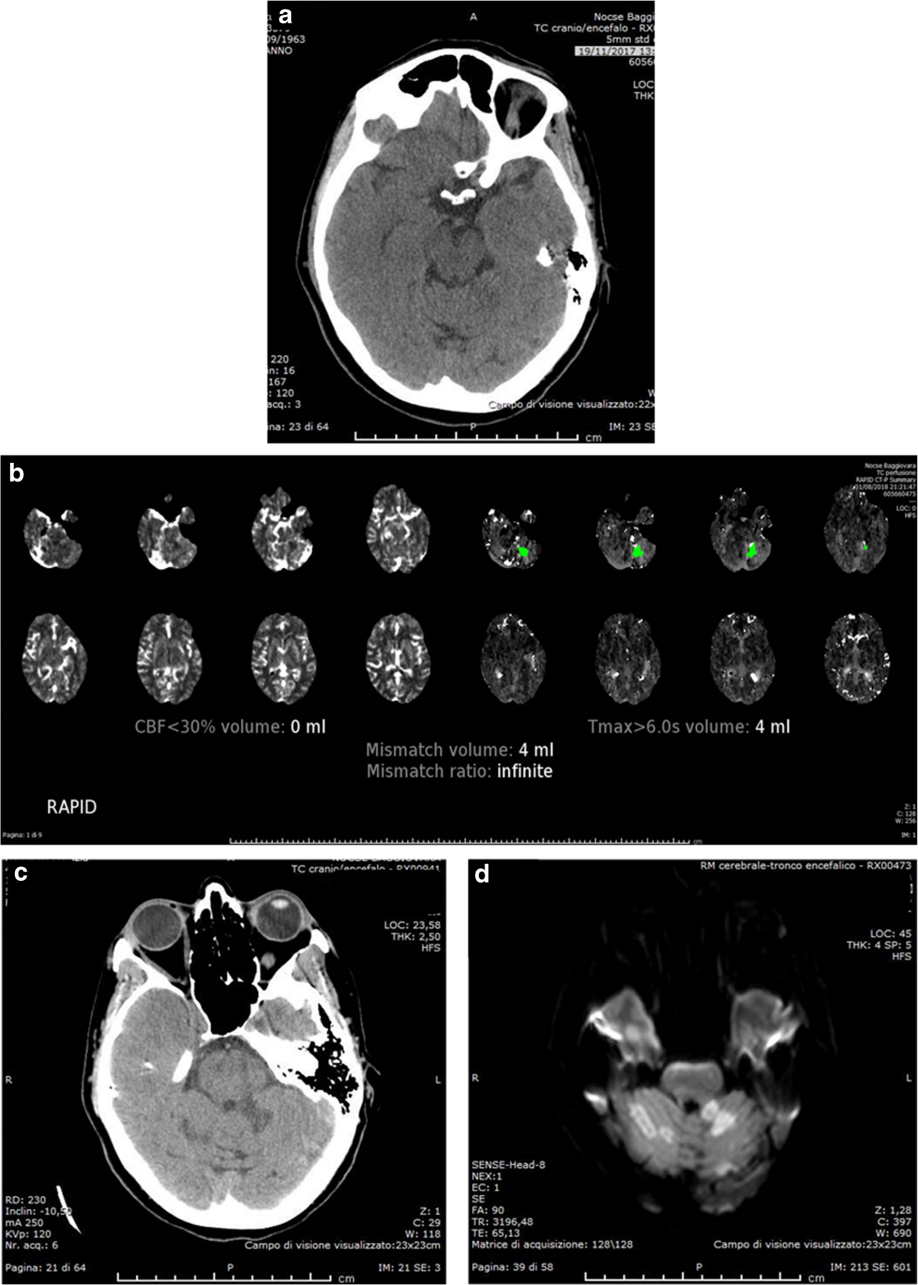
Left small cerebellar ischaemic lesion visible on initial NCCT (a) as hypodensity, not recognised as ischaemic core by the automatic RAPID mismatch map (b) but confirmed on follow-up CT (c) and DWI (d)
